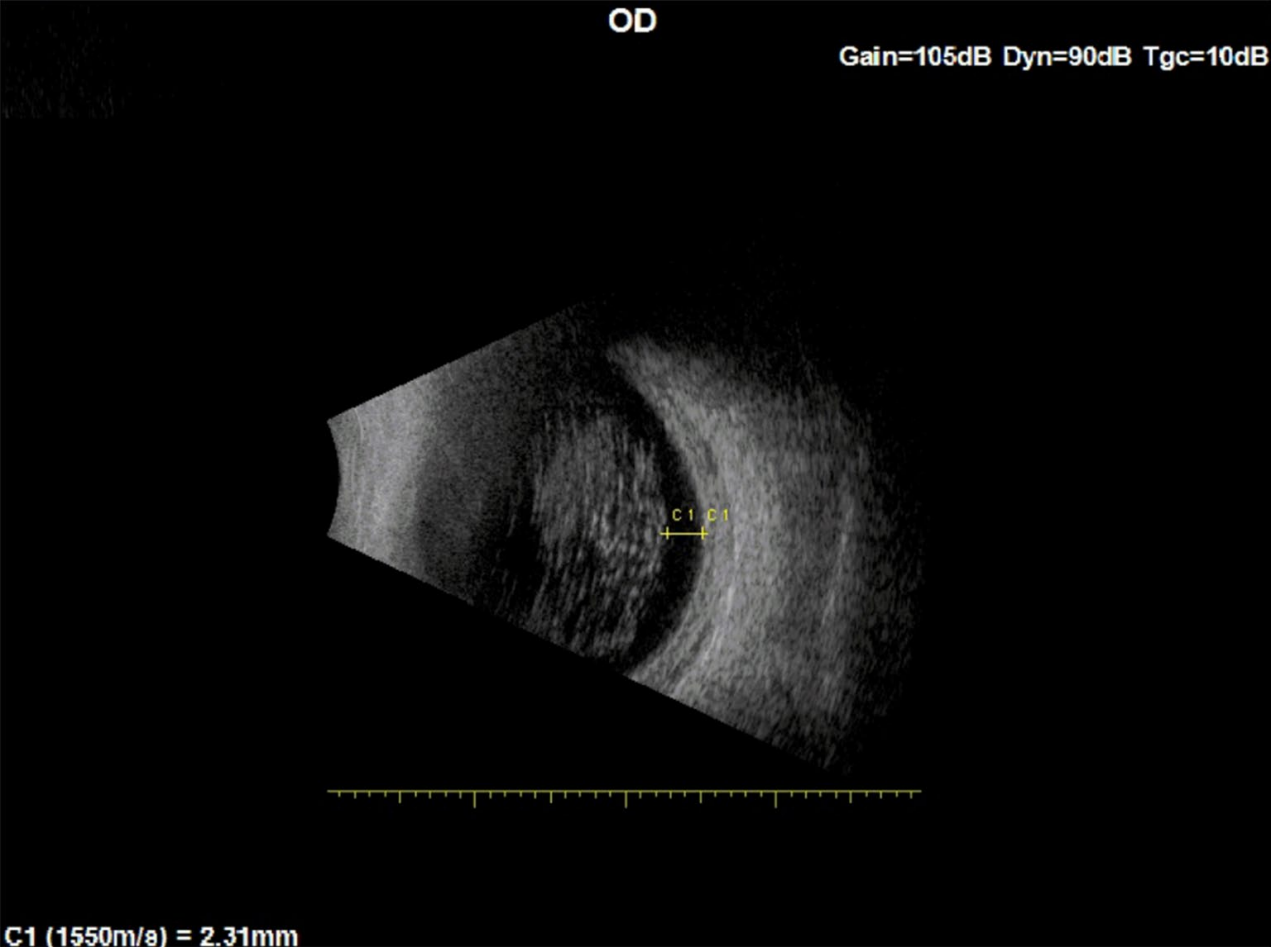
Ultrasound B-mode scan of patient 1 Right Eye. C1: measurement of the closest AH body to distance from the retina
